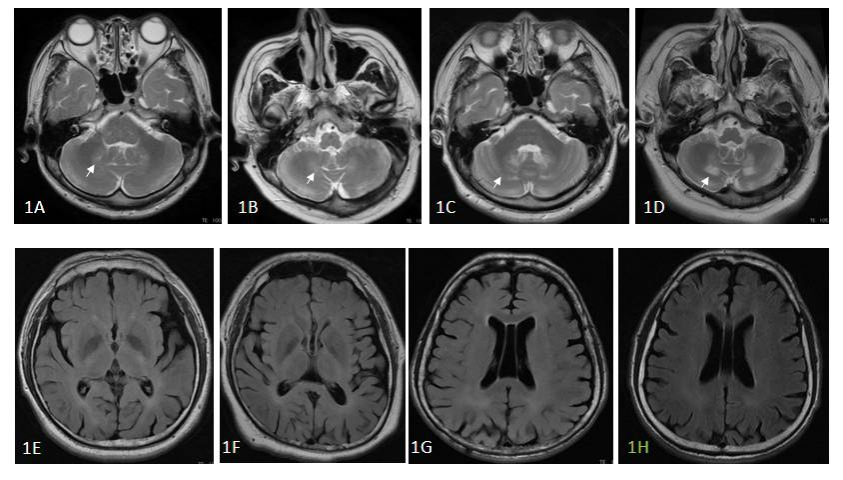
Conventional magnetic resonance images (MRI) results. MRI shows peri-dentate high signal intensities in the cerebellum in Cases 1 (1A) and 2 (1C) at diagnosis and their extension four years later (Cases 1 (1B) and 2 (1D)). Fluid attenuated inversion recovery images show equivocal high signal intensities in the peri-ventricular regions in Cases 1-4 (1E-1H).
